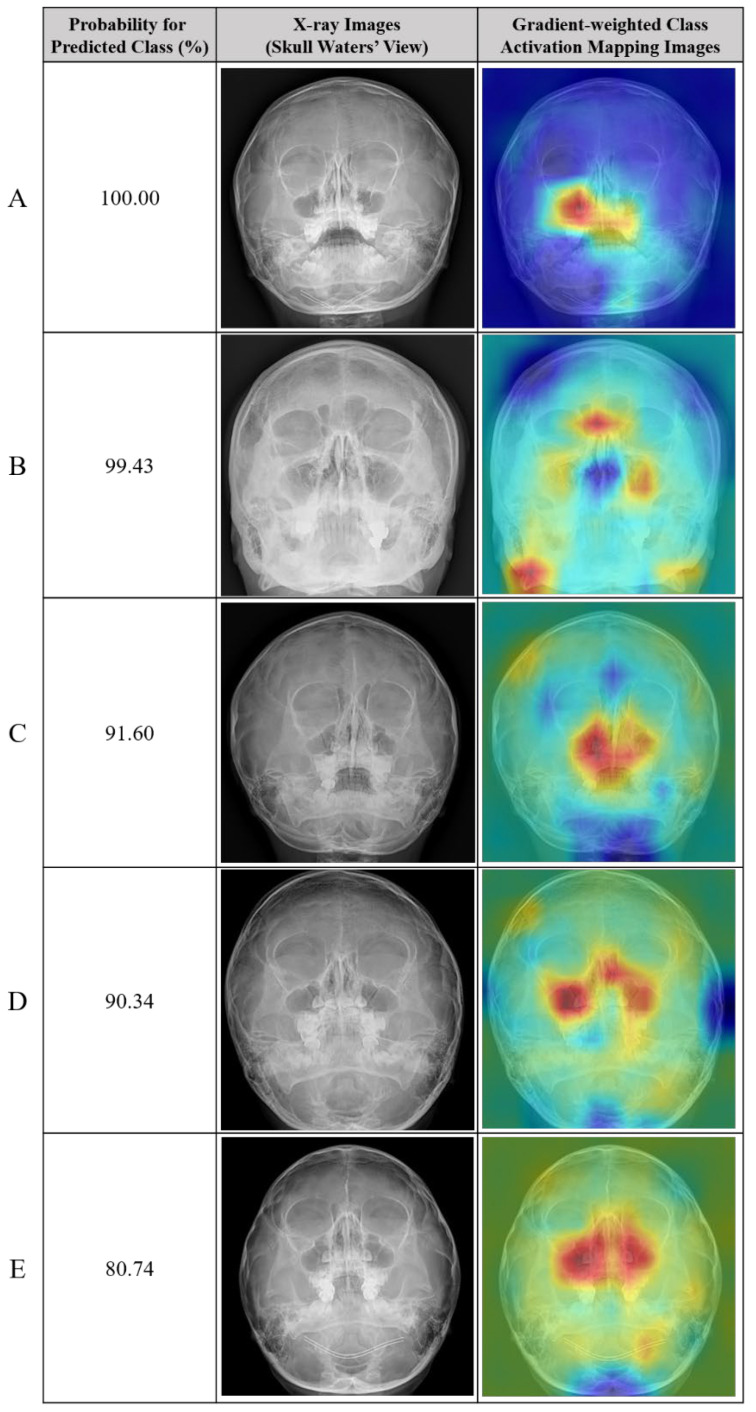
Representative cases of incorrectly predicted classes (false positive) in the test dataset using the no-code platform with a classification labeling method. (Left) Radiographic images of the Skull Waters’ view with the removal of right/left markings from the corners of the X-ray. (Right) Gradient-weighted class activation mapping (CAM) images. The probability for the predicted class (%) is also provided for each sample. (A–E) normal nasal radiographs misclassified as fractures.
